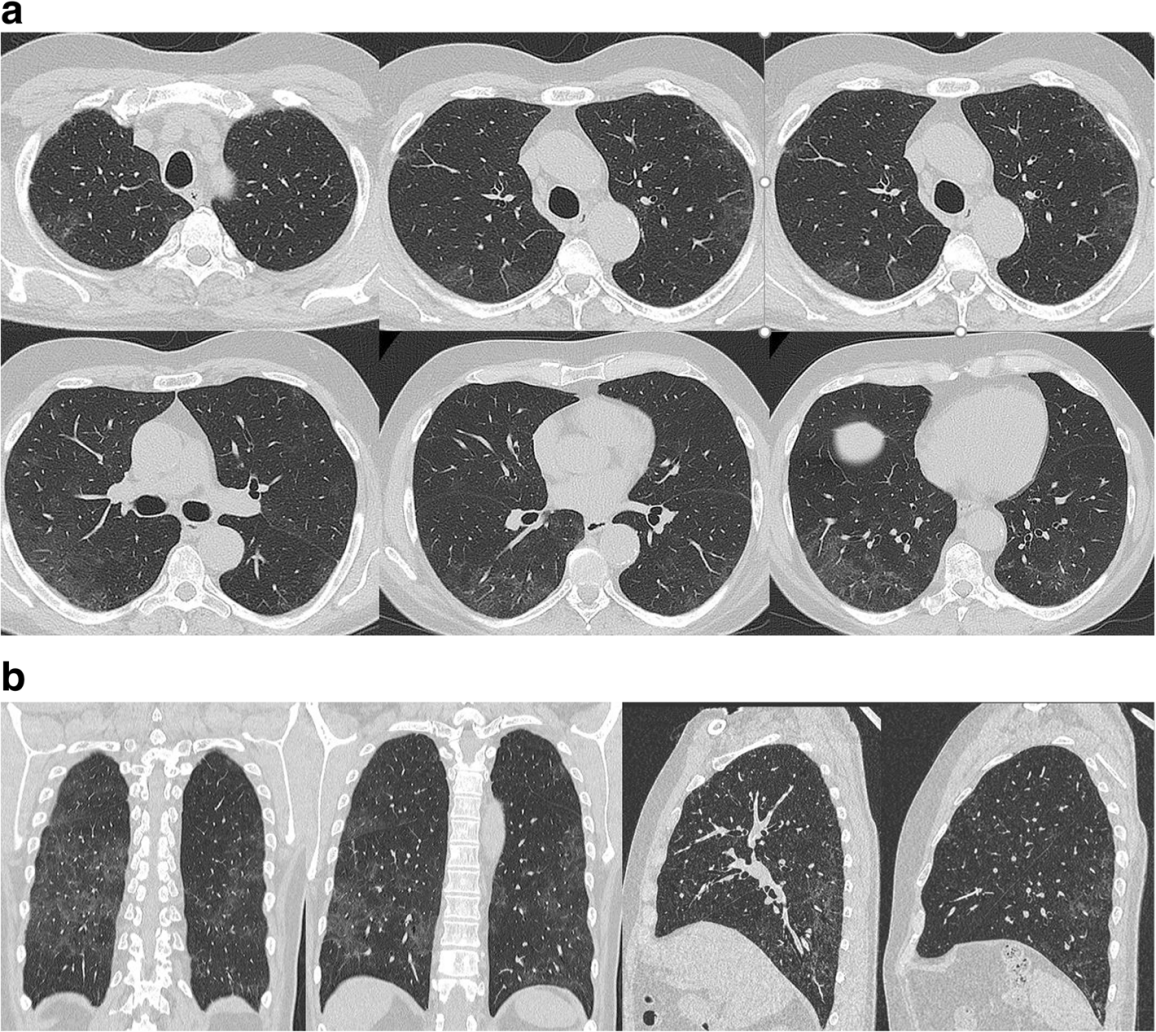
Chest CT. In (a) on axial floor. In (b) coronal and sagittal floor. There are multiple bilateral peripheral ground-glass areas, more evident at basal and right, but present at the top of lungs too associated with the crazy-paving pattern, with the prevalence of interlobular e intralobular thickening compared to the ground-glass pattern. There are no evident pulmonary consolidations and/or mediastinal lymphadenopathies
